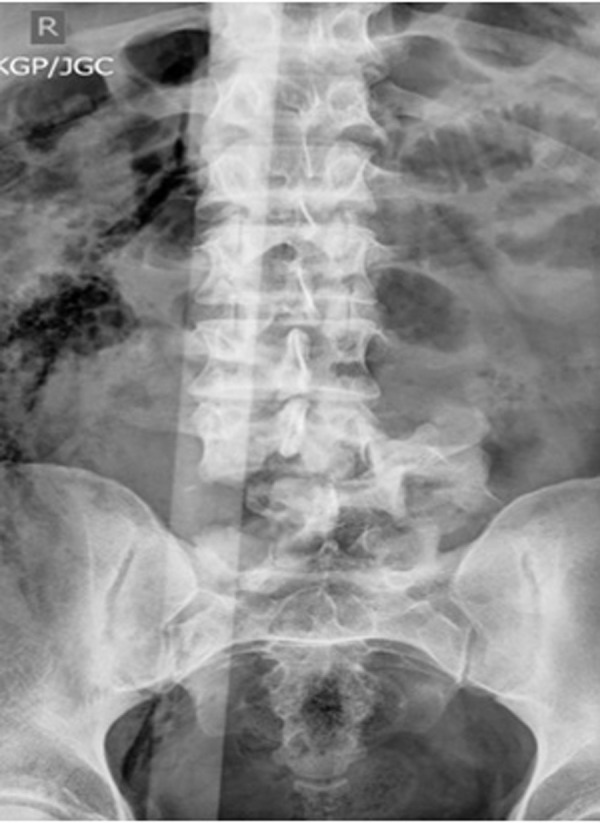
Preoperative anteroposterior x-ray This is a preoperative anteroposterior x-ray demonstrating a fracture dislocation of the L5 vertebrae relative to S1. There is significant lateral listhesis apparent.
